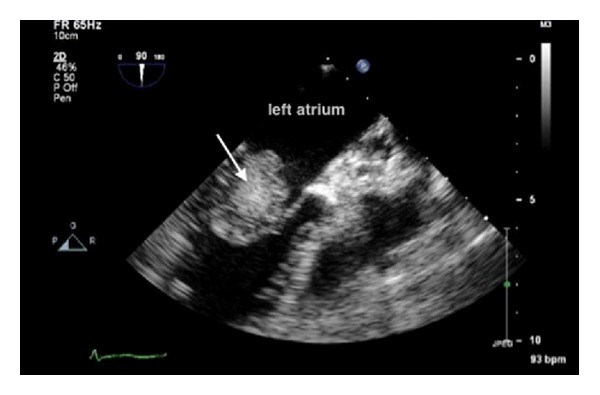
Echocardiogram showing left atrial myxoma (arrow).
